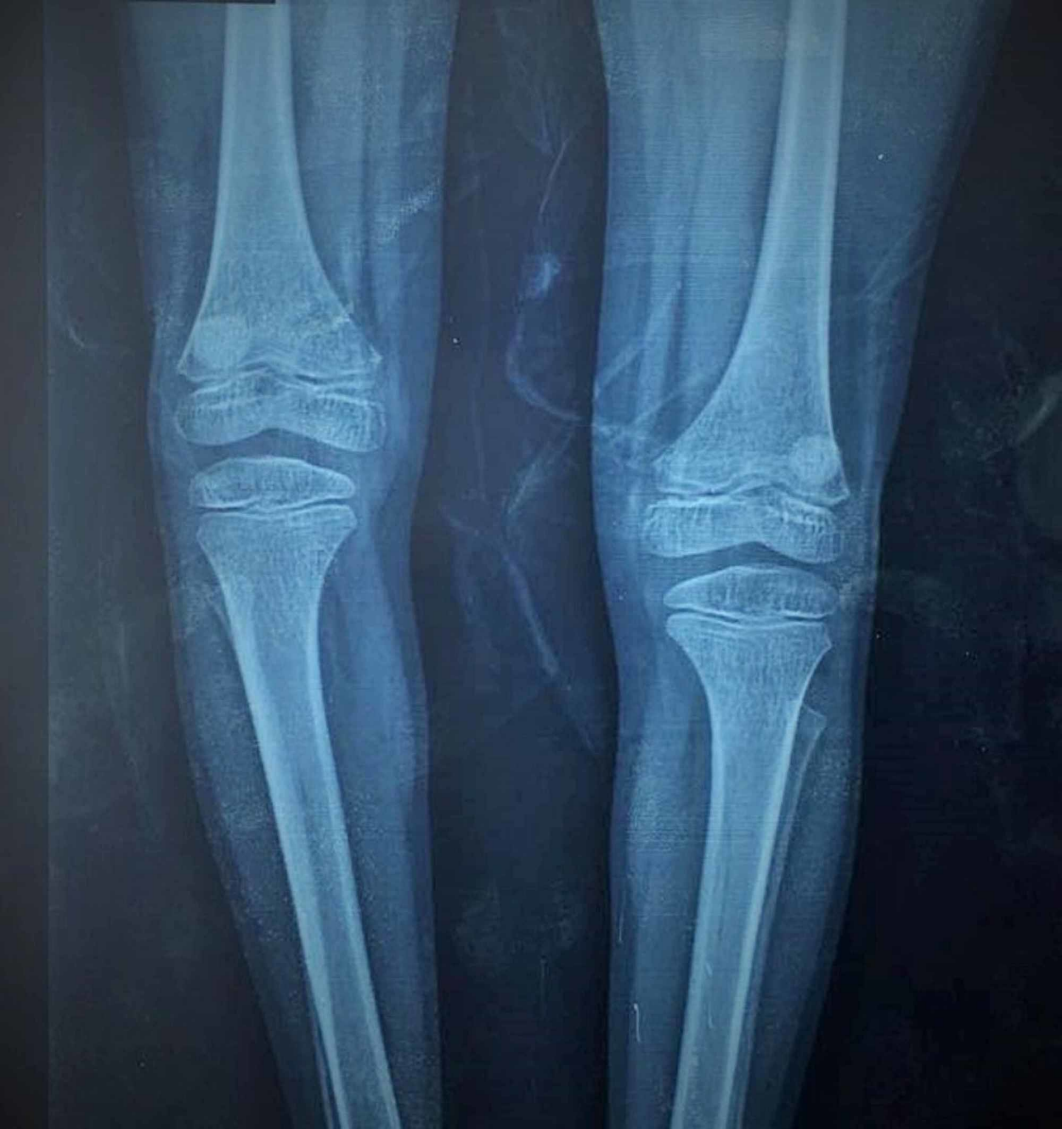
Case two: bilateral hypoplastic and subluxated patellas with varus deformity as evident on the X-ray The image indicates a very rare presentation of MOPD2, with bilateral hypoplastic patellae MOPD2: microcephalic osteodysplastic primordial dwarfism type 2
